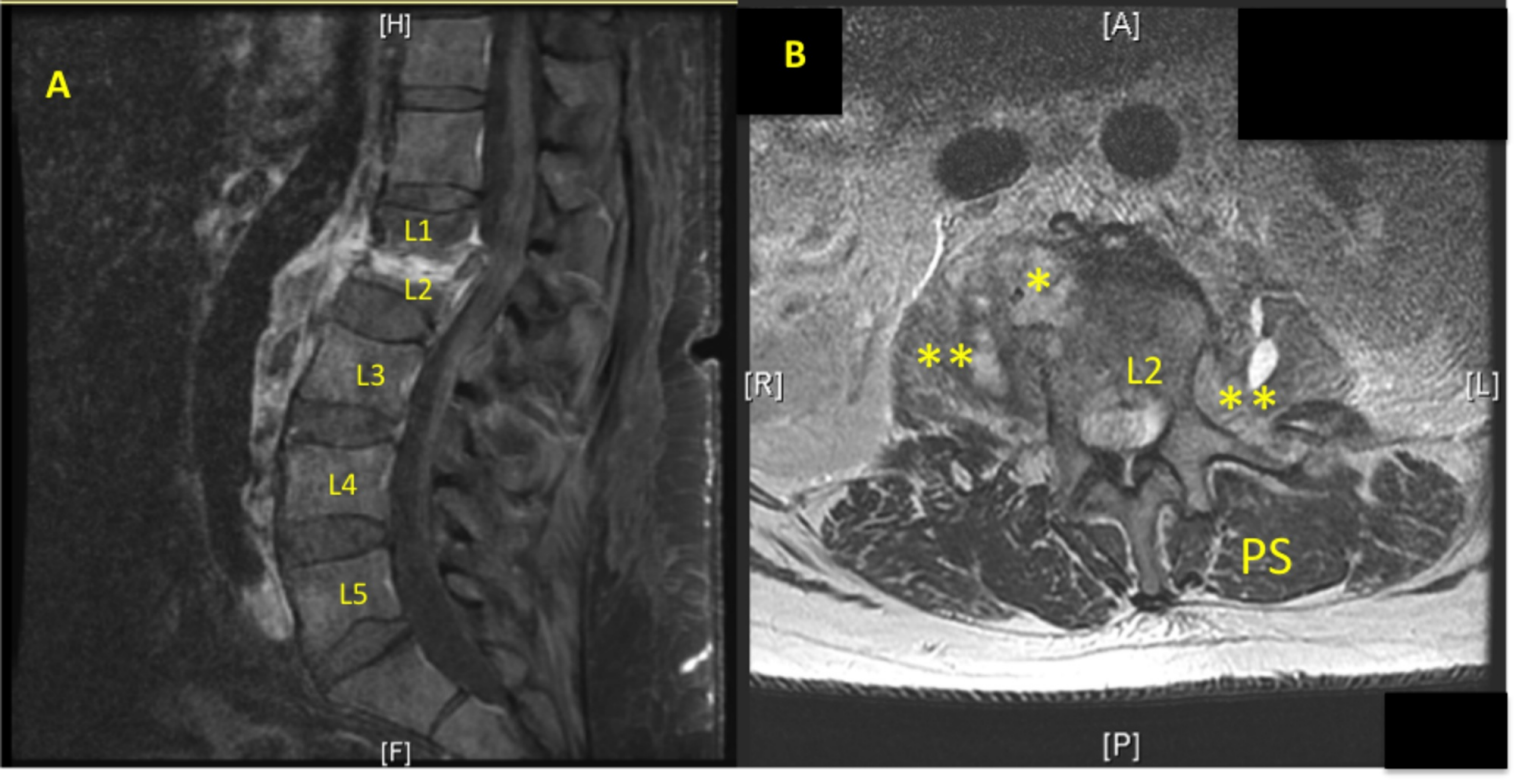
Initial Admission MRI Lumbar Initial admission, Figure 1A (MRI T1 w contrast, sagittal): Compression fracture at L2 with retropulsion of the posterior-superior margin. Bone destruction particularly involving the inferior aspect of the L1 vertebra and portions of the L2 vertebra representing discitis and osteomyelitis. Figure 1B (MRI T2 wo contrast, axial): Paravertebral phlegmon (yellow *) extending into left and right psoas muscles (double yellow *) with fluid signal intensity suggesting small abscesses. Moderate ventral thecal sac compression and moderate spinal stenosis. Paraspinal muscles (PS).
